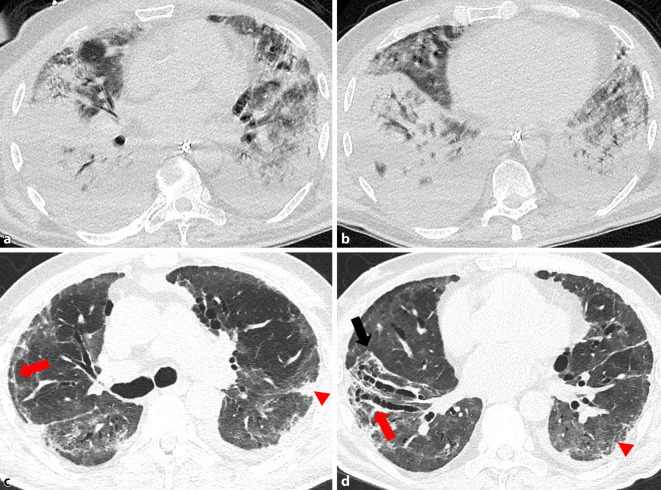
Neben den oben beschriebenen pulmonalen Veränderungen ist eine COVID-19-Erkrankung auch ein Risikofaktor für die Entwicklung thrombotischer und embolischer Ereignisse. Diese Ereignisse können ebenfalls mit längerfristigen Veränderungen assoziiert sein. Die Dual-Energy-CT (DECT) ermöglicht die Beurteilung der pulmonalen Perfusion und die Erkennung kapillarer mikrovaskulärer Thrombosen [1]. Die DECT-Angiographie 3 Monate nach COVID-19-Pneumonie zeigte in einer Studie bei 5,4 % der Patienten eine proximale arterielle Thrombose und bei 65,5 % der Patienten Perfusionsanomalien, die auf eine weit verbreitete Mikroangiopathie hinwiesen. Vier Patienten mit normalem Lungenparenchym zeigten eine gestörte Mikrozirkulation der Lunge [29].
